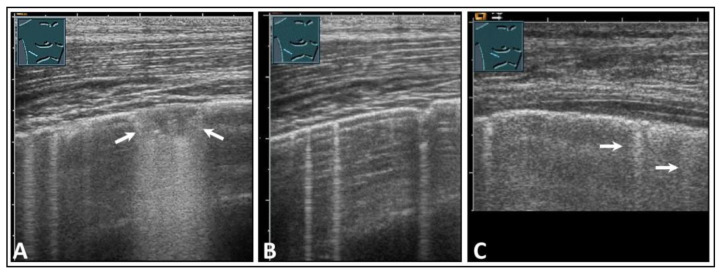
A patient with clinically diagnosed infectious pleuritis who presented with right-sided respiratory-dependent chest pain. (A) On lung ultrasound, a consolidation of approximately 5 mm with dorsal sound enhancement (arrows) was observed, along with (B) multiple B-lines (arrows), as well as (C) isolated comet-tail artifacts (arrows) with pleural thickening and a delicate effusion.
